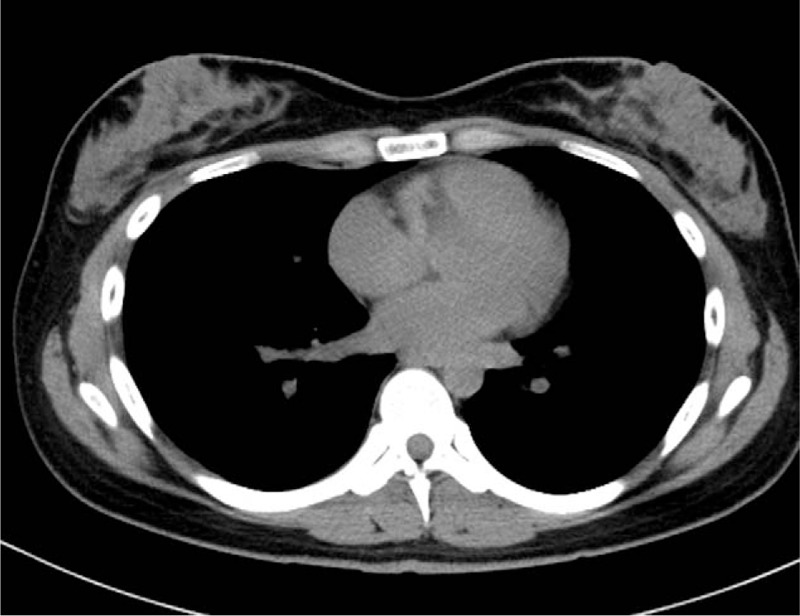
Chest plain CT. The right pleural effusion disappeared and diffuse right pleural thickening improved.
